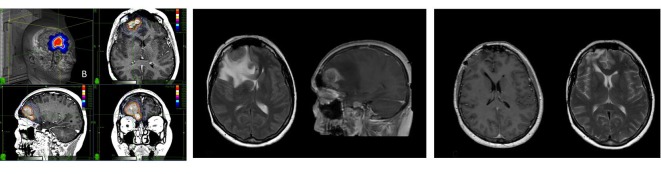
Single fraction CyberKnife radiosurgery treatment of a right frontal meningioma causing symptomatic post-treatment edema (PTE) with resolution after surgical resection of the tumor. Left: Right frontal meningioma radiosurgery treatment plan (single fraction; prescribed dose 13 Gy) in a patient affected by meningiomatosis. The patient had undergone 5-session (25 Gy) radiosurgery for a petroclival meningioma one year before. Middle: Seven months after the treatment, the patient presented with confusion and seizures progressing to status epilepticus. The MRI showed perilesional edema causing frontal lobe compression with midline shift. Right: The patient underwent resection of the frontal meningioma with quick resolution of edema and symptoms. Of note, the treatment of the skull base tumor did not cause any complication.
